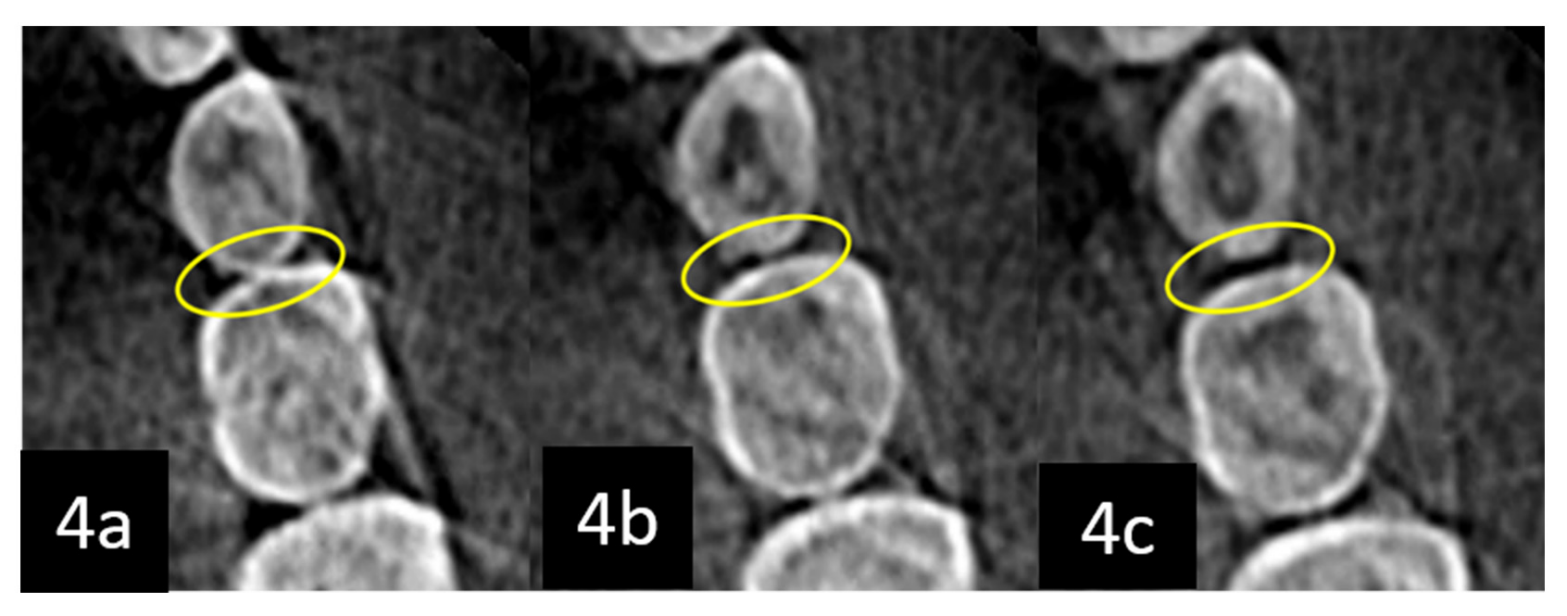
CBCT images of the interproximal contact areas of the primary molars in the mandible. The (
